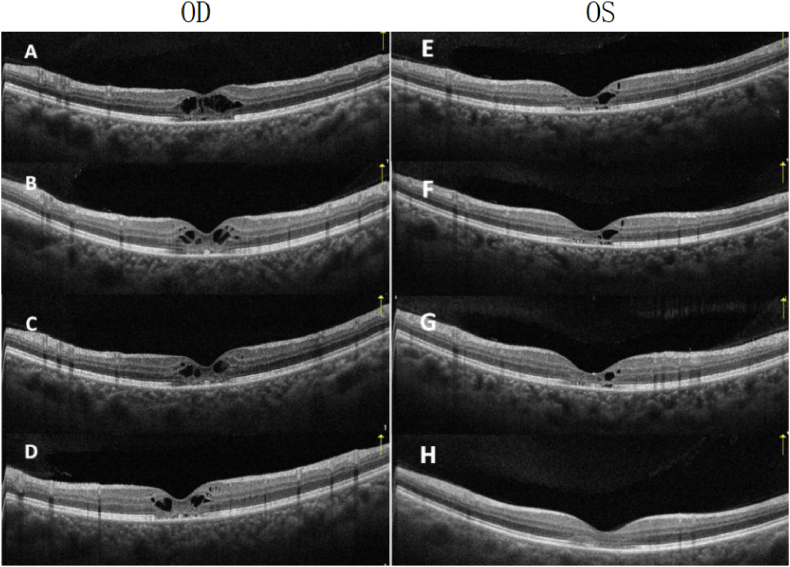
OCT changes in both eyes. (A,E) Baseline examination. Intraretinal cystic changes in fovea, mainly affect IPL and OPL with discontinuity of outer retinal layers. (B) Changes at one month after cessation of loratadine; macular cystic changes partially resolved. (C) Two months after cessation of loratadine; continued resolution of intraretinal cysts and outer retinal layers. (D) Six months after cessation of loratadine; residual intraretinal cysts still observed in OD. Complete restoration of intraretinal layers and partial recovery of outer layers in OS. IPL, inner plexiform layer; OPL, outer plexiform layer; OCT, ocular computed tomography.
